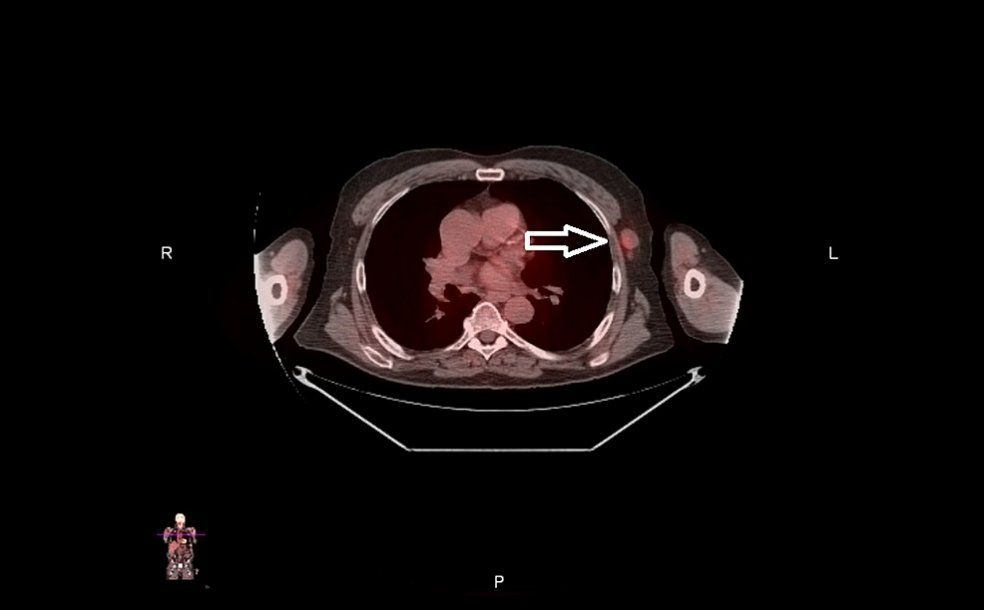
Axial view of PET-CT scan showing moderate FDG uptake in the lymph node of the left axillary/subpectoral region (white arrow). PET: positron emission tomography; FDG: fluorodeoxyglucose
